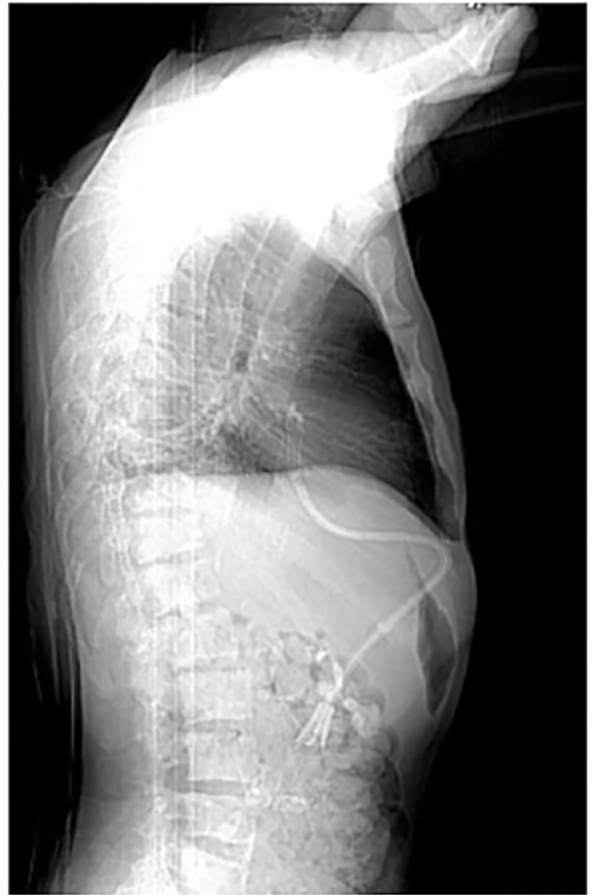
Post-procedure radiograph showing the positioning of the transhepatic hemodialysis catheter with its inner tip in the right atrium.
